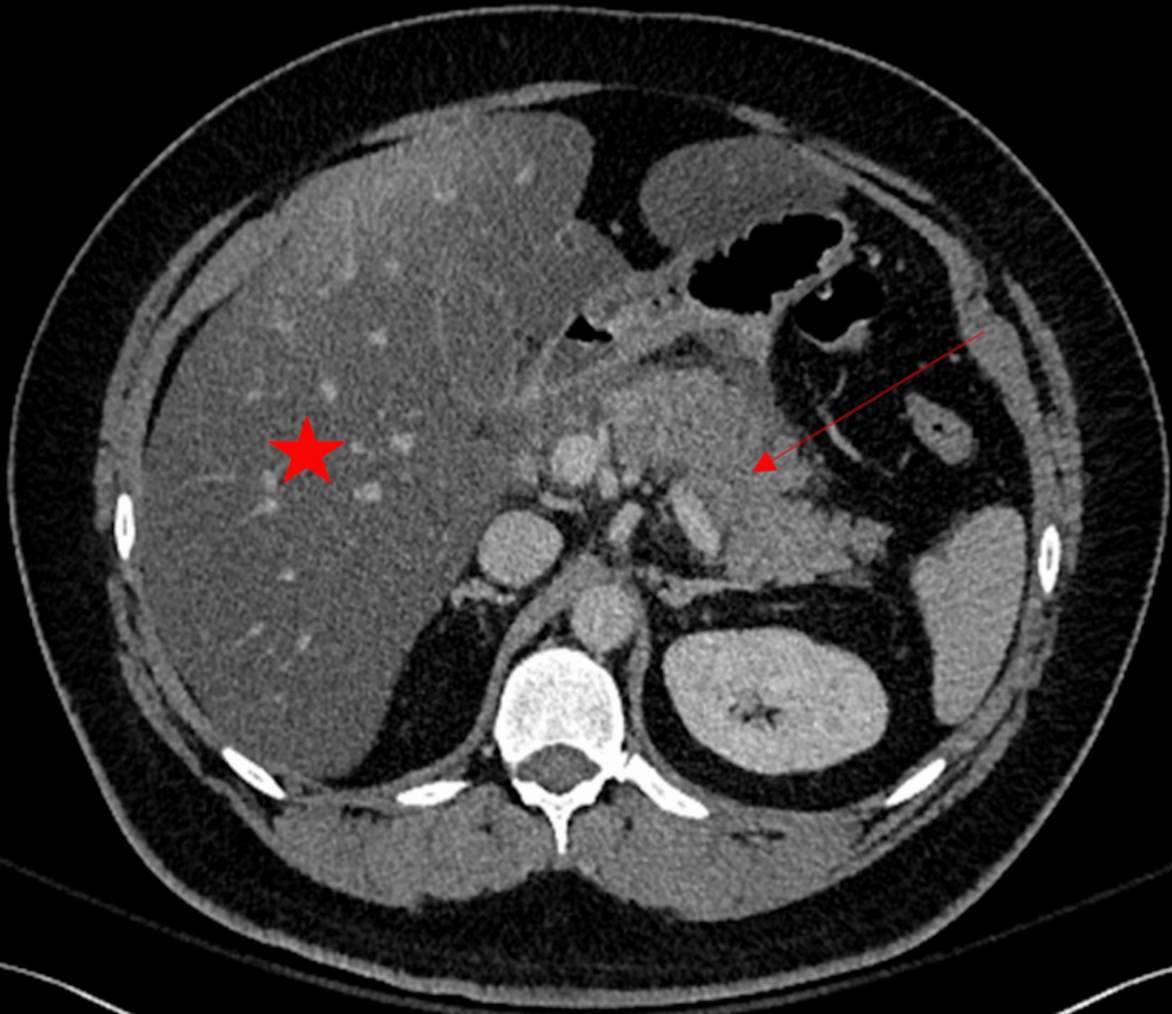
CT scan of the abdomen with contrast. The image demonstrates diffuse hepatic steatosis (star) and substantial fat stranding as well as fluid extending into the bilateral paracolic gutters (arrow). Fat stranding and fluid accumulation can extend into the bilateral paracolic gutters, consistent with findings in acute pancreatitis.
